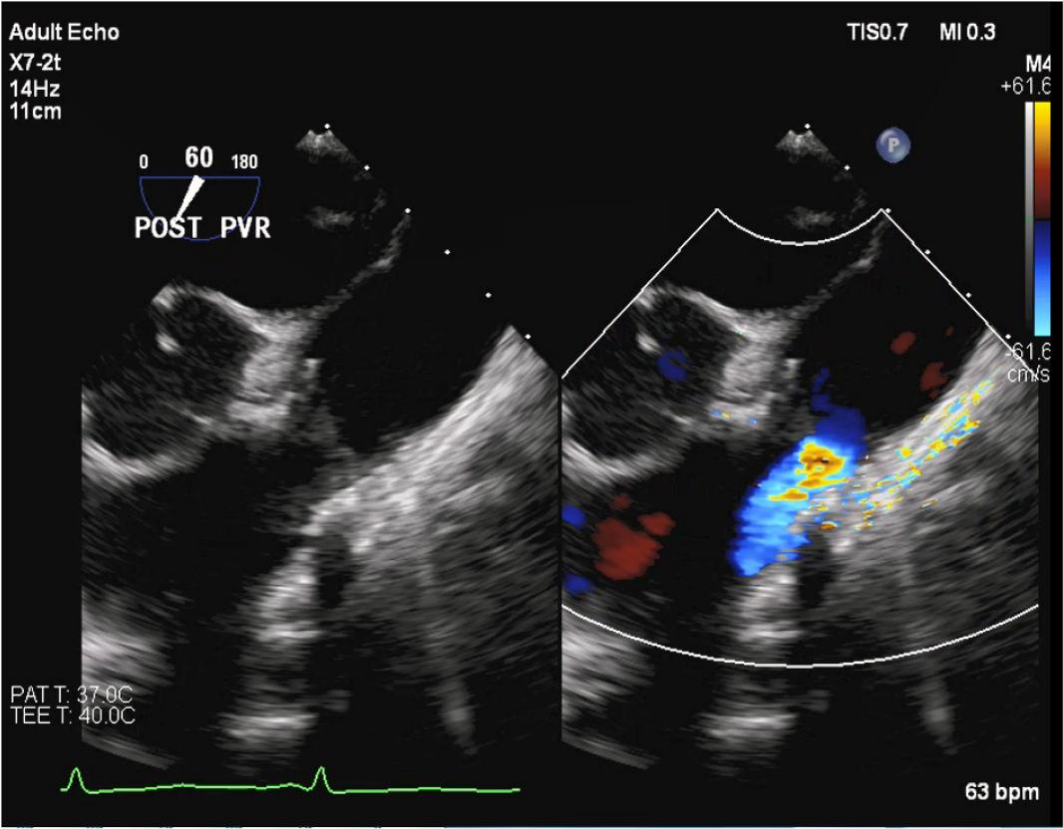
Intra-operative trans-oesophageal echocardiography, mid-oesophageal short-axis view at 60°, showing reduction in the pulmonic regurgitation to mild post-transcatheter valve-in-valve intervention.
